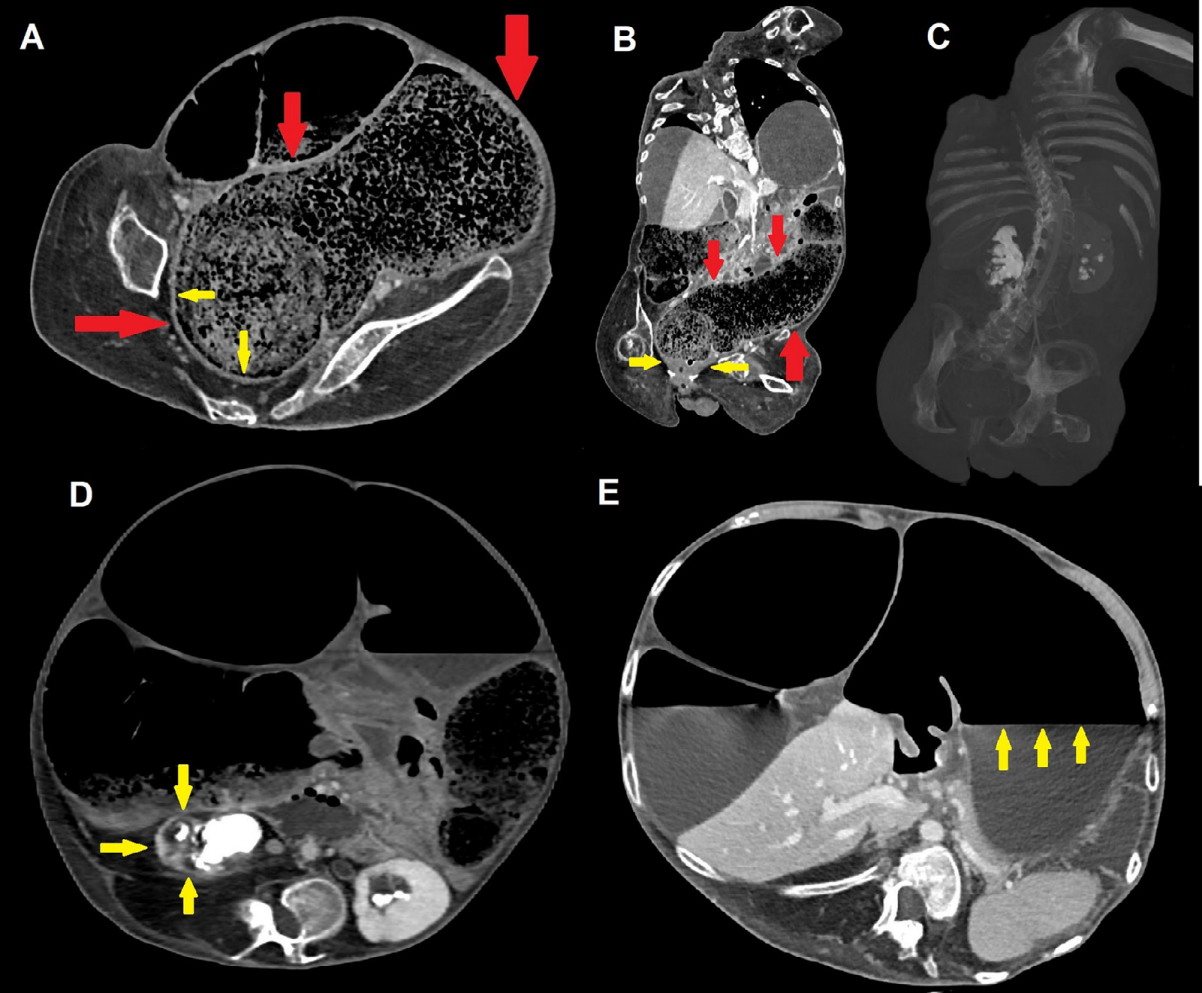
Axial (A, D-E) and coronal (B, C) contrast-enhanced CT scans demonstrate an extensive recto-sigmoid fecaloma measuring more than 30 × 10 cm (red arrows); chronic thickening of rectal walls is visible (yellow arrows), as well as compressive bilateral grade III hydronephrosis with staghorn stones and thinning of renal parenchyma, frequently seen in DMD patients (C and D, yellow arrows). Dilatation with atonia and an evident air-fluid level also involves the stomach (E, yellow arrows), further witnessing the multifocal nature of gastrointestinal motility disorders in patients with DMD.
